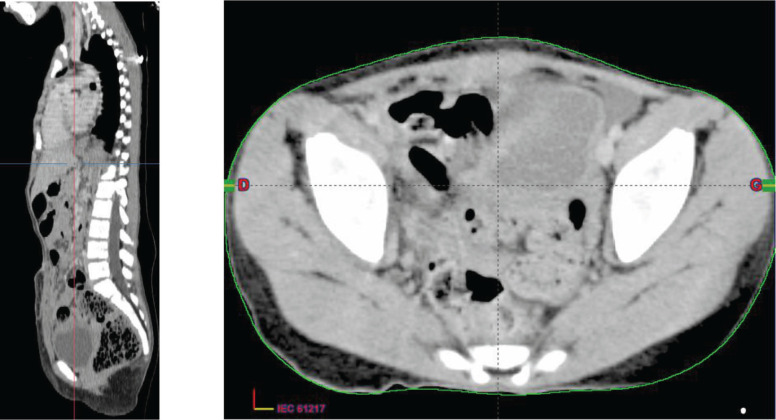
Chest–abdomen–pelvis postoperative CT scan showed the absence of any residual tumour.
